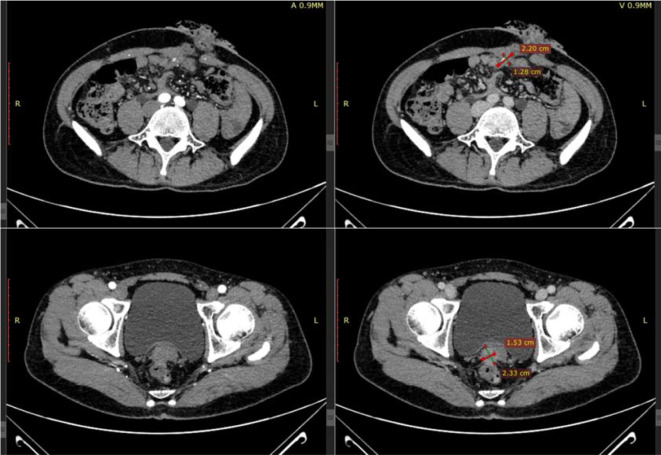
Three months after T-DXd treatment, abdominal CT in May 2023.
